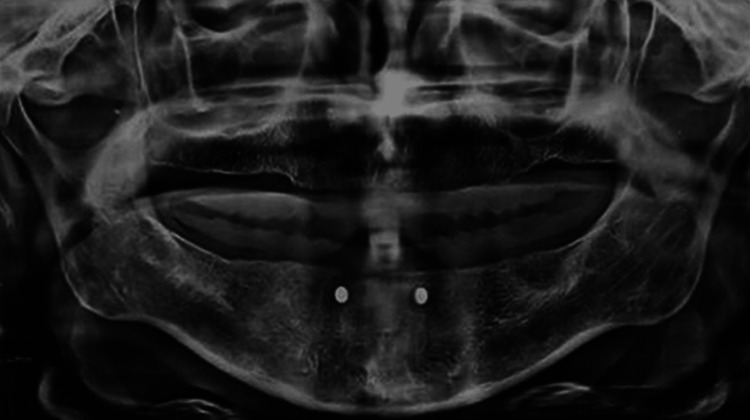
OPG with placement of steel balls. OPG, orthopantomogram
